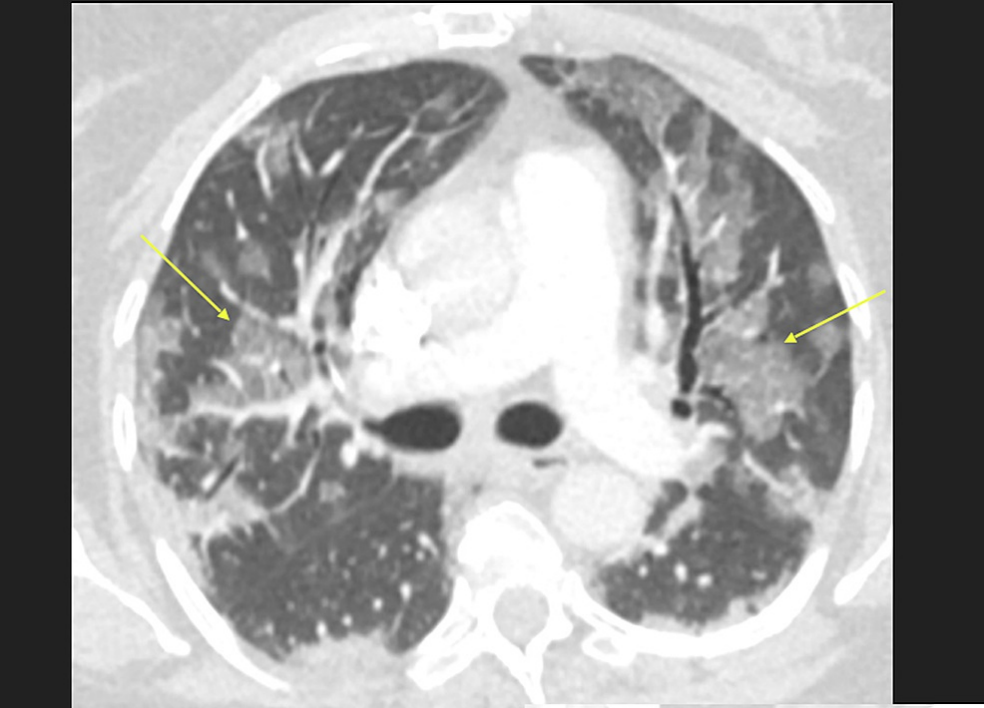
Axial CT image shows bilateral ground glass opacities (arrows) with involvement of all five lung lobes.
